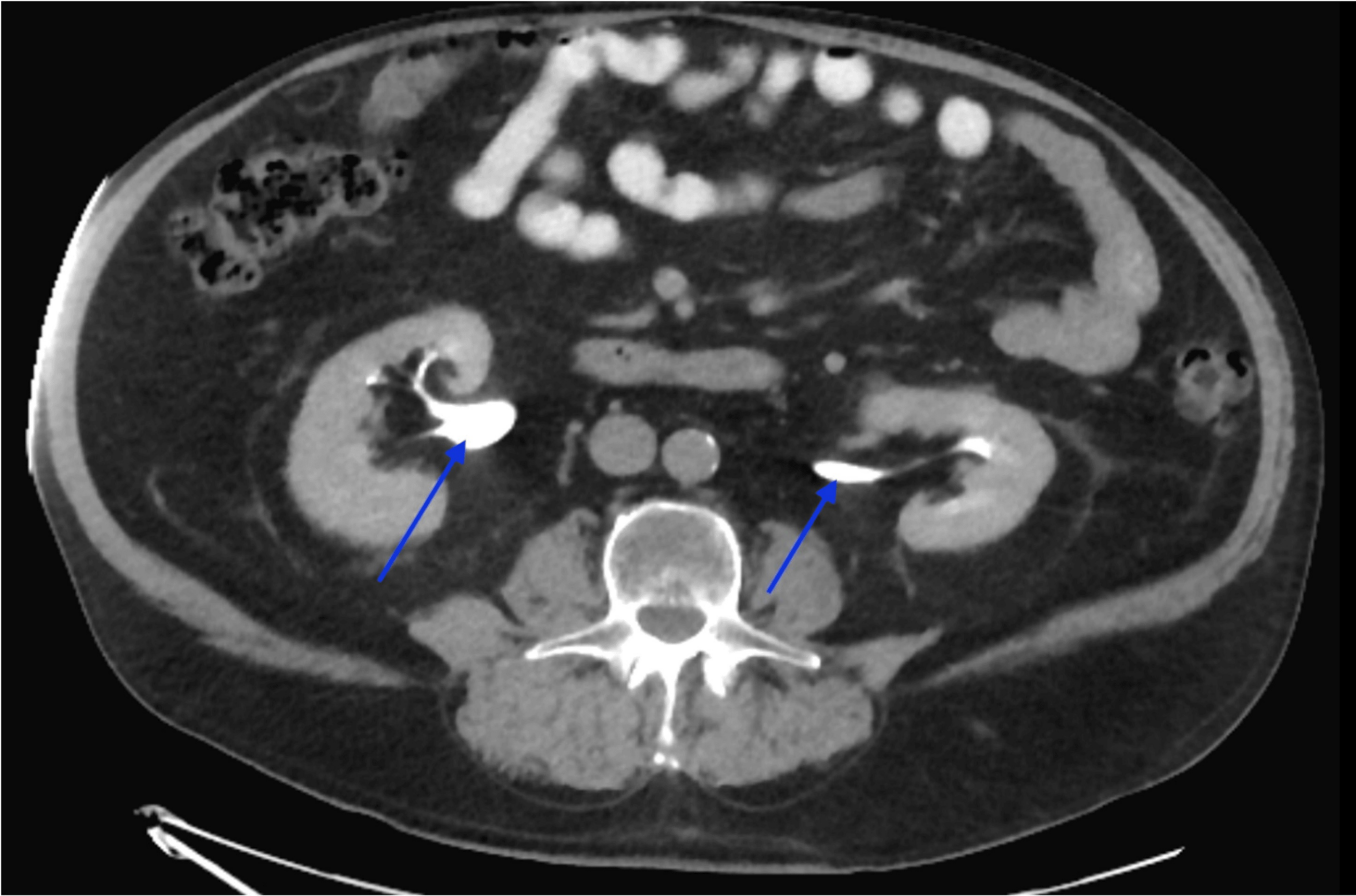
CT with contrast showing the ureters’ origin (blue arrows) at the patient's renal pelvis.
